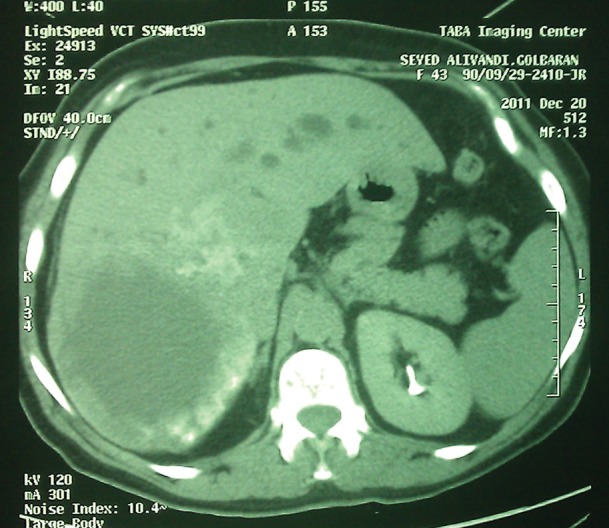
CT Scan Shows Well Defined Masses in Right Lobe of the Liver
